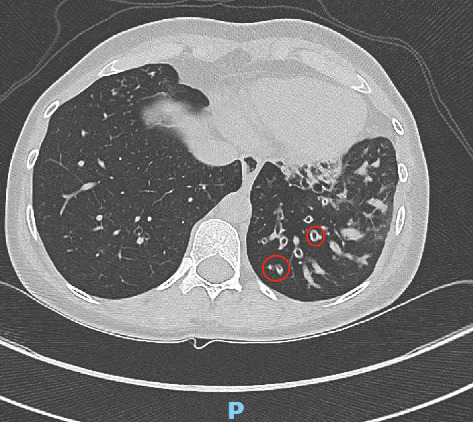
This image shows more severe bronchiectasis seen in the lower lung fields in this patient. Bronchiectasis is more severe in the lower lungs than the upper, as is typical in PCD as compared with CF, where the upper lobes are usually more severely affected. Bronchiectasis is noted radiographically when the diameter of the bronchus exceeds the diameter of the accompanying bronchial artery, as in the two pairs with red circles.
